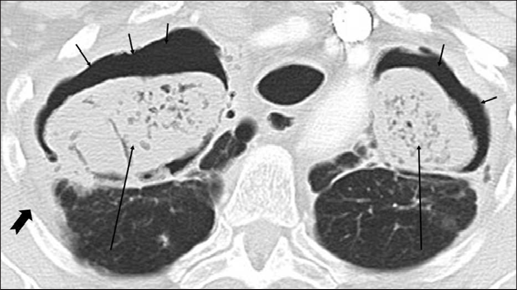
Aspergilloma.* A 58-year-old male sarcoidosis patient also had known long-standing fibrosis. Routine CXR revealed opacities in the apical segments of both lungs. CT showed large content known fibrotic cysts apically with crescent-shaped air (short arrows) anteriorly due to large formed fungus balls (long arrows). There was local pleural thickening (thick arrow) (no symptoms).
